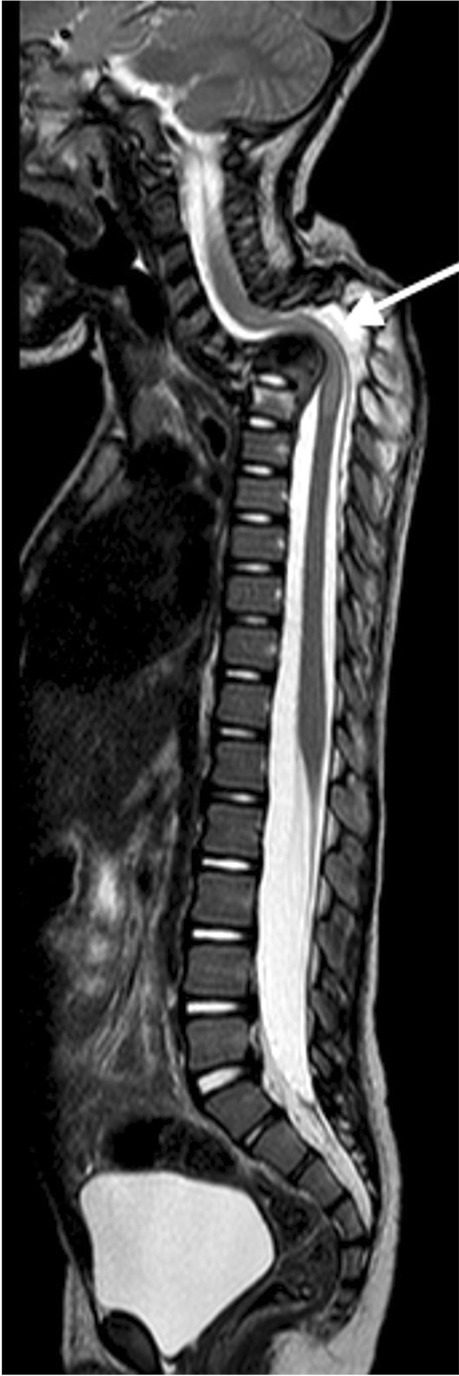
Sagittal T2 magnetic resonance image in a 4-year-old boy presenting with kyphosis and tetraparesis. There is cervicothoracic kyphosis and the cord is draped over the apex of the gibbus with localised cord signal abnormality (*arrow*). The patient made a full recovery post-surgical and anti-tuberculous therapy
